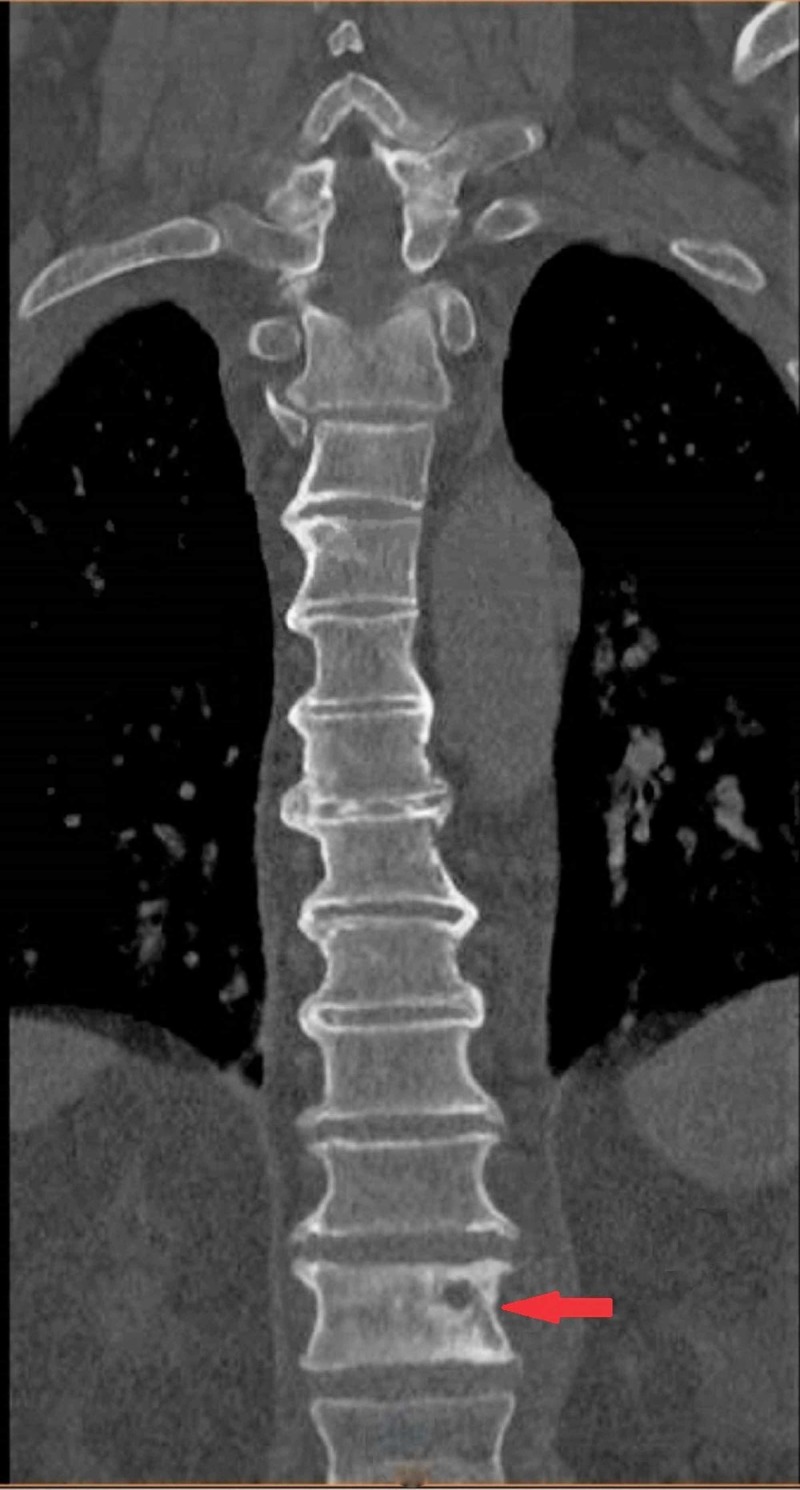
MRI lumbar spine coronal section demonstrating the involvement of the L3 vertebra
